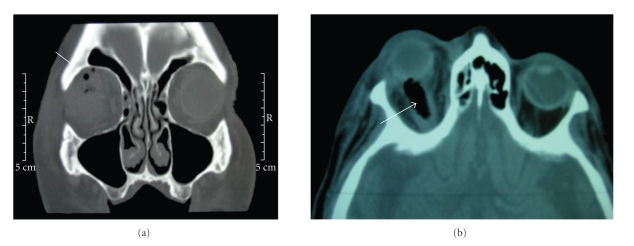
CT at the admission. (a) The arrow indicates extraconal air bubbles, (b) intra-conal air bublle.
